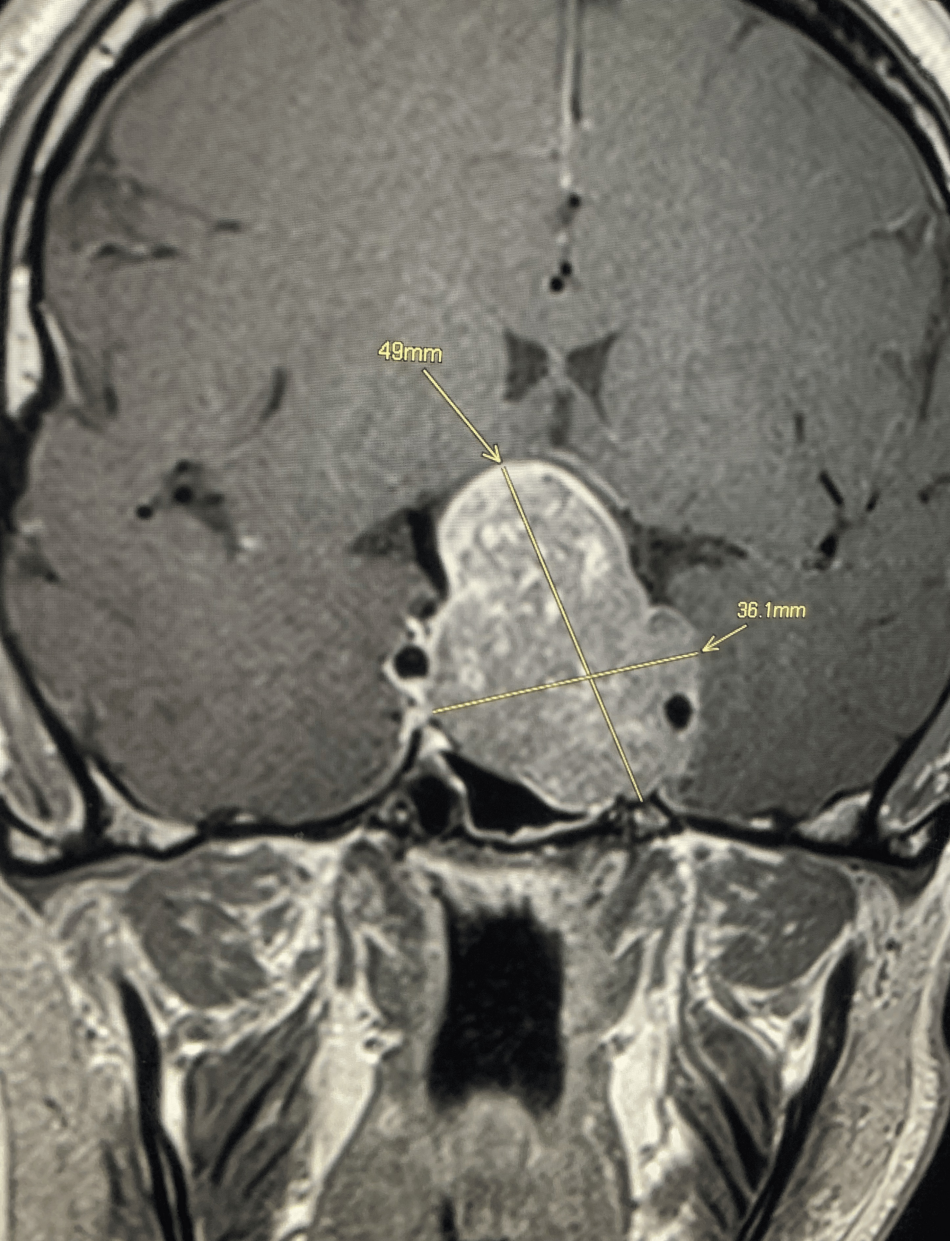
Pituitary MRI - coronal view Before the treatment, showing the initial dimensions of the lesion.
